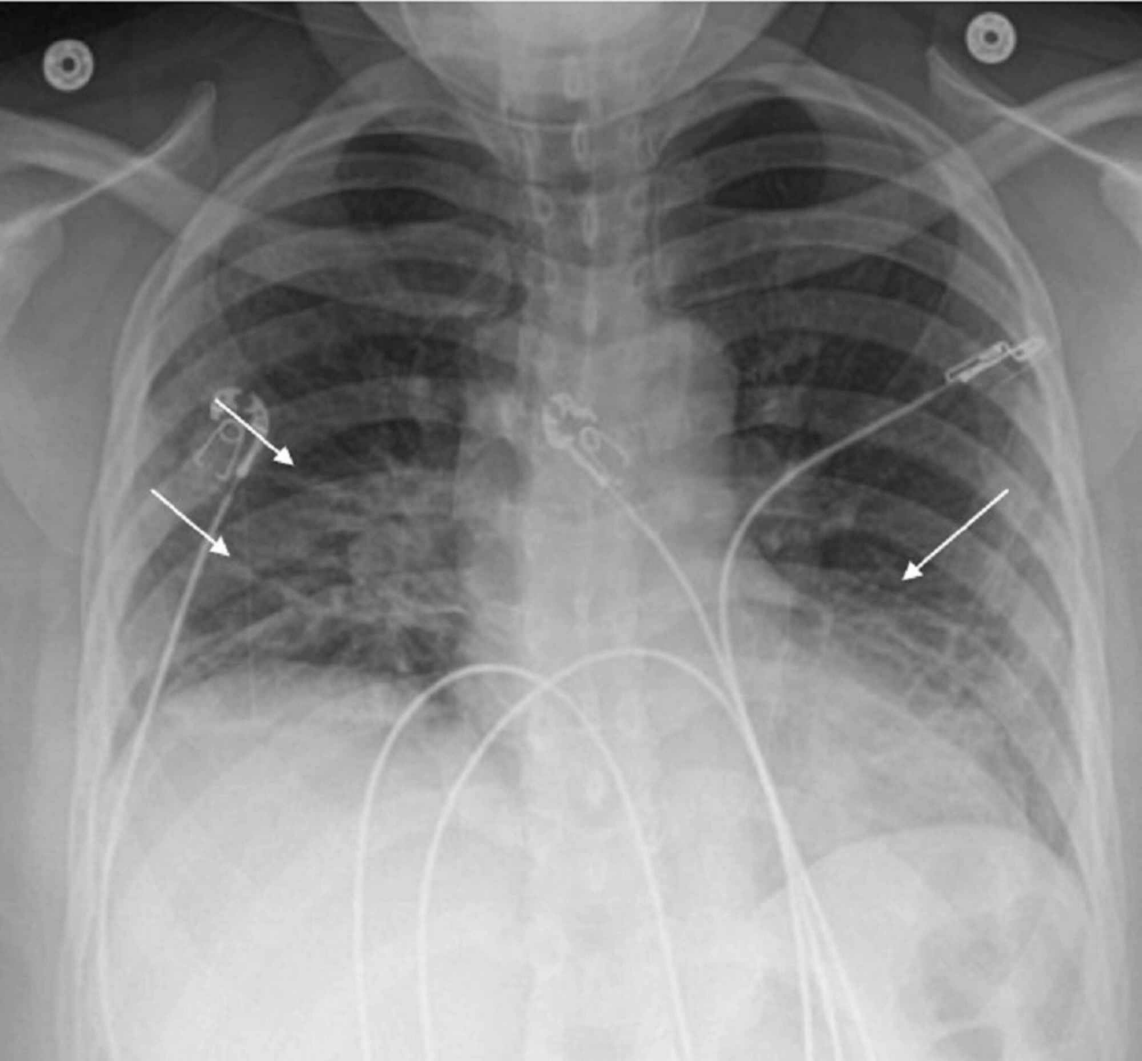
Chest X-ray on admission showing bilateral interstitial infiltrates predominantly in the lower lung fields.
